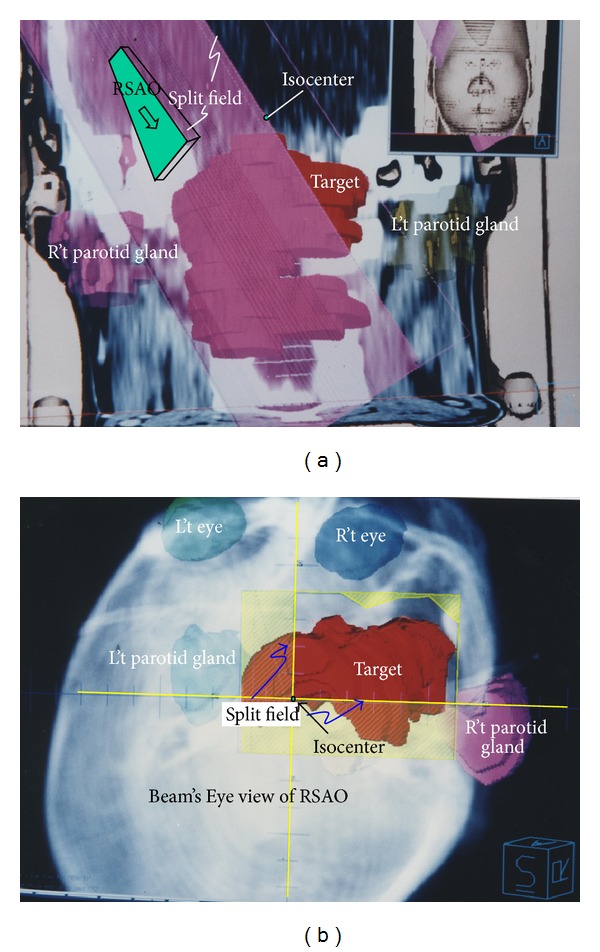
(L't) Picture shows the split field of right superior anterior oblique (RSAO) field in coronal plane. The red color wash area in the center of this picture represents the target. Right and left side parotid glands were represented in pink and brown color, respectively. Both right and left side parotid glands were spared with block. In this field, couch was rotated to 120°, gantry was rotated to 260°, and collimator was rotated at degree of 180°. 60-degree wedge with “thick head” toward gantry side was added for dose uniform modification. (R't) Picture shows the Beam's Eye View (BEV) in Digital Reconstruction Radiograph (DRR) of RSAO field. RSAO beam shape (yellow color) covers most of the lesion. There is two-field edge with no divergence, horizontal and vertical field edge along the central axis plane. Both right and left side parotid glands were spared by asymmetry closing jaw.
